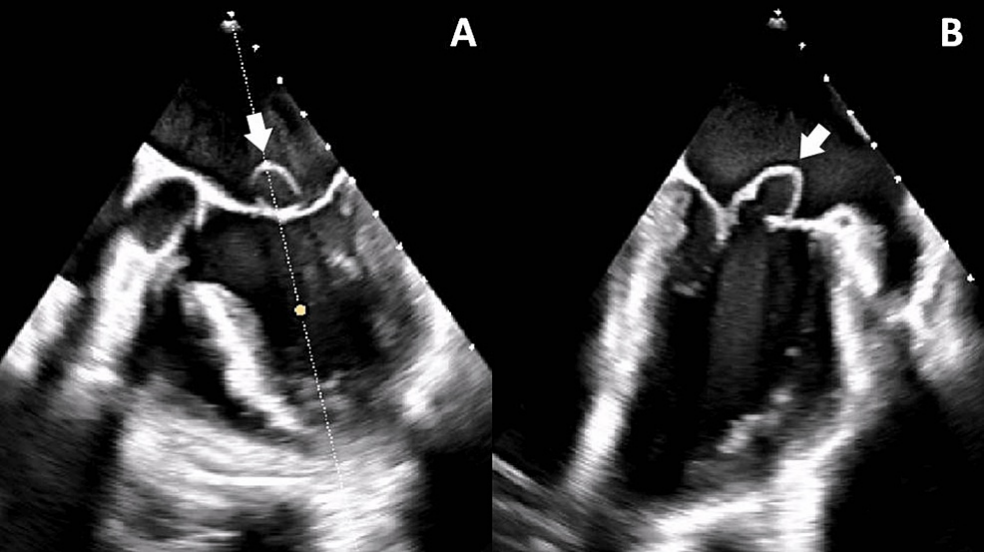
(A, B) Transesophageal echocardiogram revealing a pseudoaneurysm of the anterior mitral valve leaflet (arrows)
